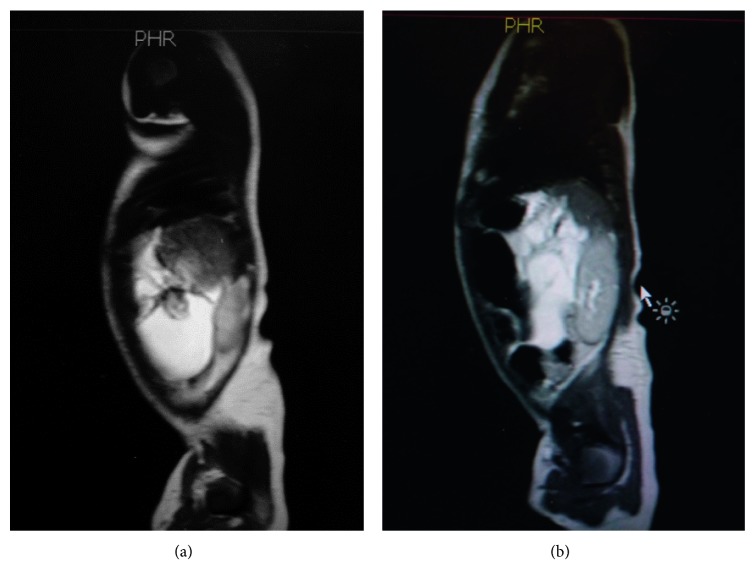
Postnatal MRI depicts the enlargement of the lesion.
